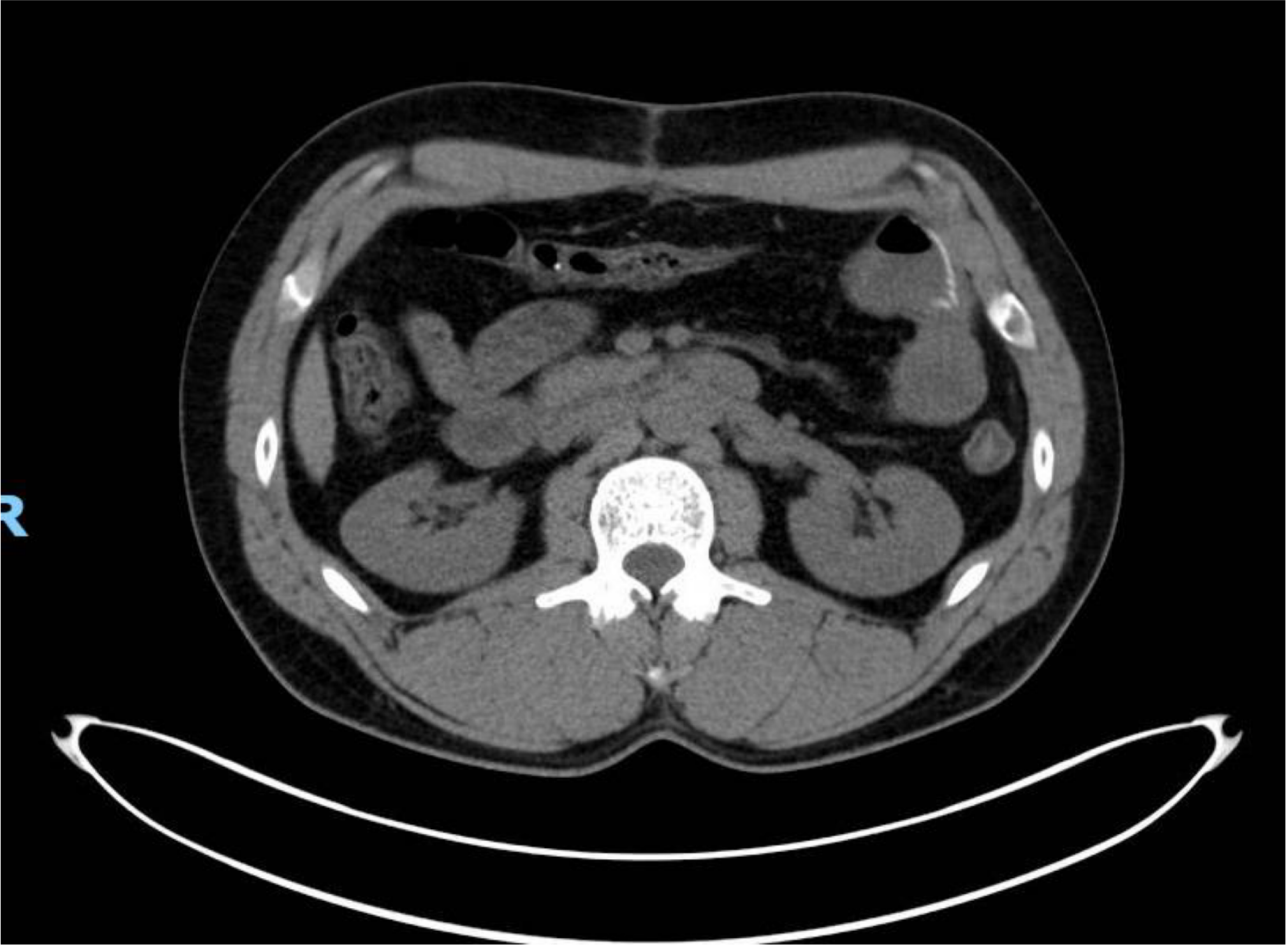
Positron emission tomography (PET) scan obtained after proximal to mid-jejunal resection and reinitiation of imatinib in July 2024. PET scan was notable for complete resolution of previous FDG-avid intra-abdominal masses seen in the CTA of abdomen in Figure 2.
